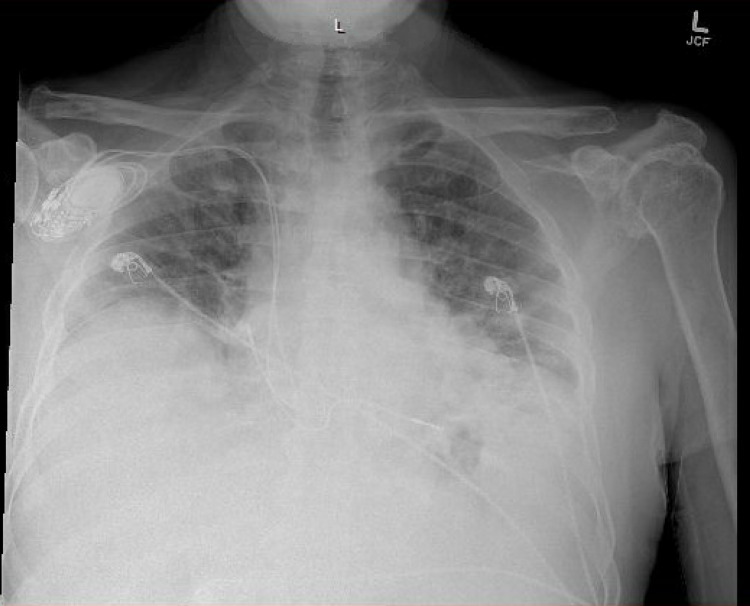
Chest X-ray on day one showing increased interstitial lung markings
